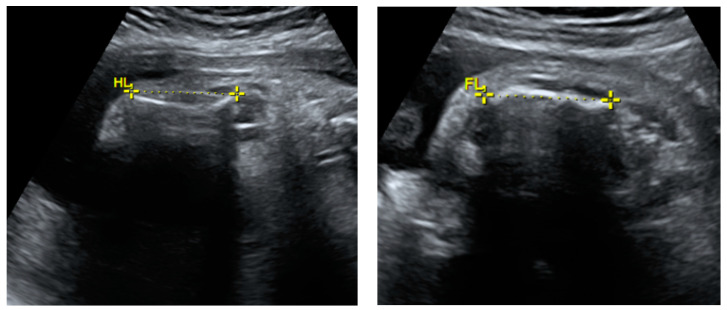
Transabdominal fetal ultrasound scan images including fetal long bones. Deformed and shortened long bones (humerus and femur) were observed. HL—humerus length; FL—femur length.
